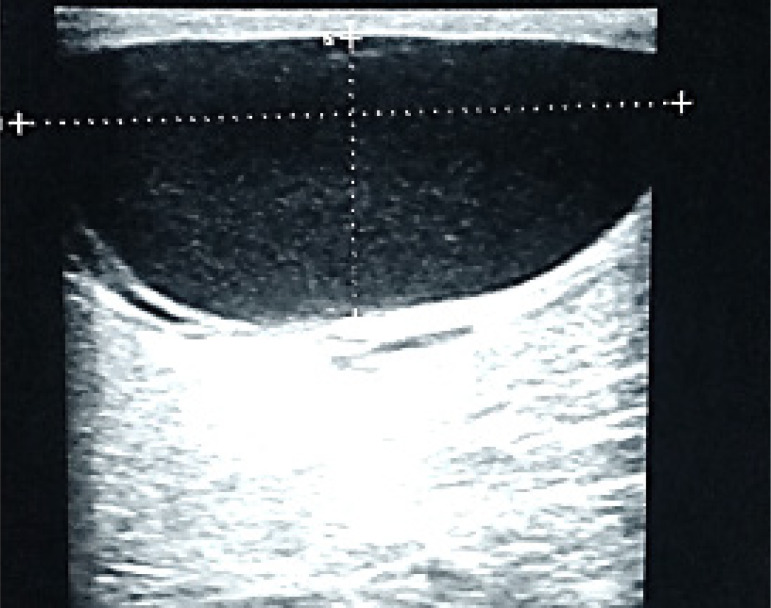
Complicated cyst A 35-year-old lactating female who complained of a non-tender firm mass in her left breast Ultrasound scan showing an oval shaped anechoic mass with moving, homogenous, echogenic internal material. Needle aspiration yielded milk Diagnosis: Galactocele
